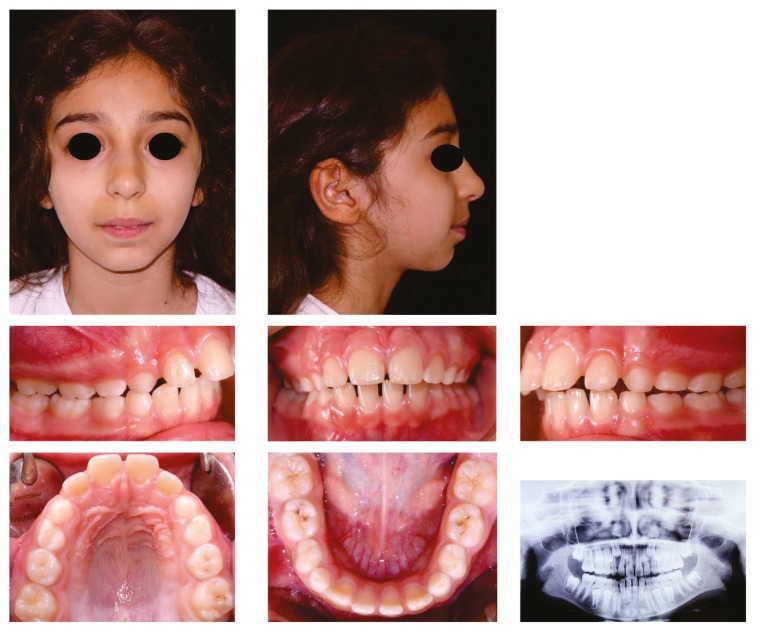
Full orthodontic records of one female patient affected by juvenile idiopathic arthritis (JIA). Extraoral and intraoral pictures show a concave profile and bilateral Angle Class II relationship. The panoramic radiograph suggests advanced degeneration of left and right condyles.
